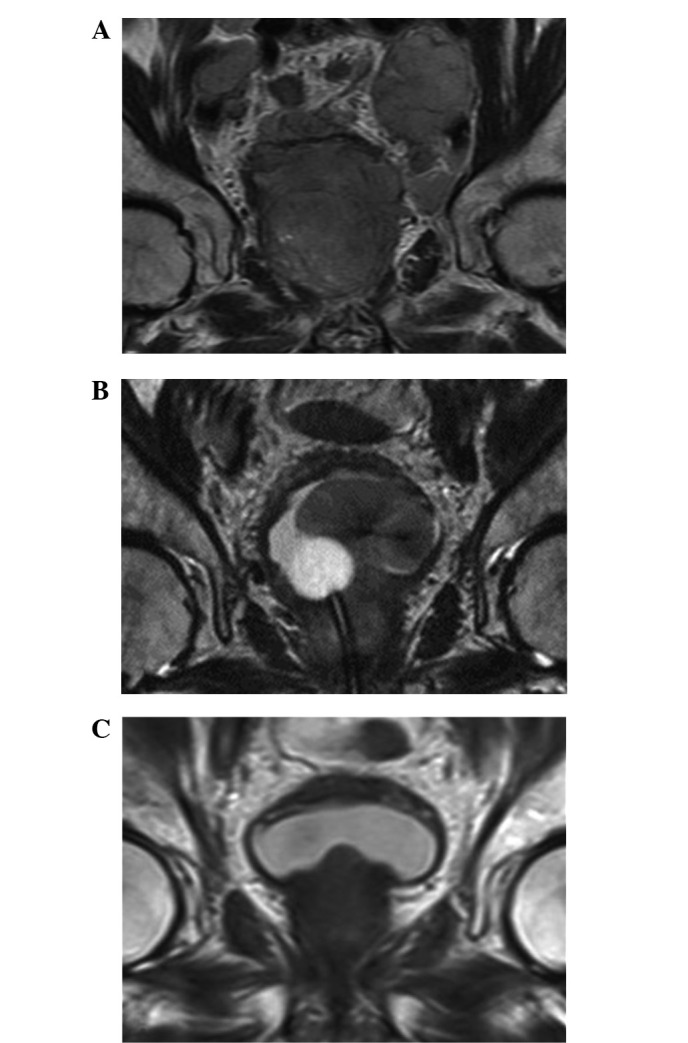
(A) Pelvic MRI obtained prior to the androgen-ablation therapy demon strating the presence of an invasive prostatic tumor and numerous large metastatic LN lesions. (B) Pelvic MRI following the androgen-ablation therapy demonstrating a locally advanced prostatic tumor, which projected into the bladder and had reduced LN swelling. (C) Pelvic MRI following the combination treatment involving EBRT and intra-arterial infusion chemotherapy demonstrating that the prostatic tumor had markedly diminished. LN, lymph node; EBRT, external-beam radiotherapy.
